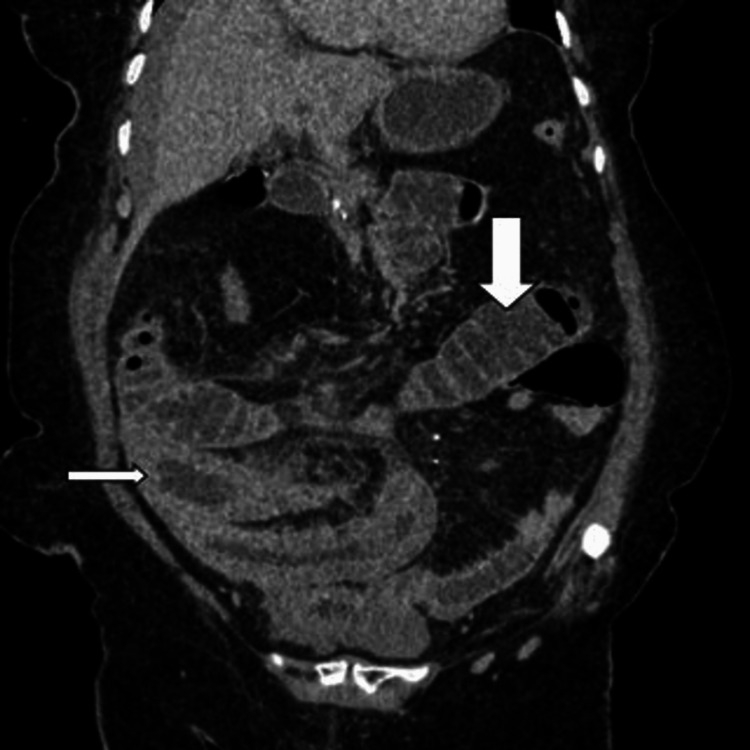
A contrast CT in a coronal slice shows a parietal hematoma (narrow arrow) responsible for a bowel obstruction (wide arrow) with the infiltration of the mesenteric fat with reactionary circumferential parietal thickening of some grelic gloves.
